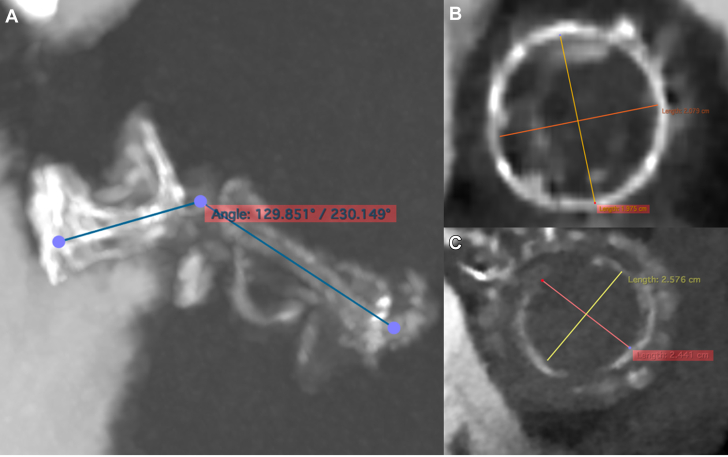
Preprocedural Screening Using Nonsynchronized CT Scan Acquired in the Emergency Department (A) The aorto-mitral angle of 129° reduced the risk of left ventricle outflow tract obstruction. (B) The Trifecta 23 true internal diameter of 21 mm was confirmed on the pulmonary computed tomography (CT) scan. (C) The Biocor Epic 29 true internal diameter of 25 mm was confirmed on the pulmonary CT scan.
